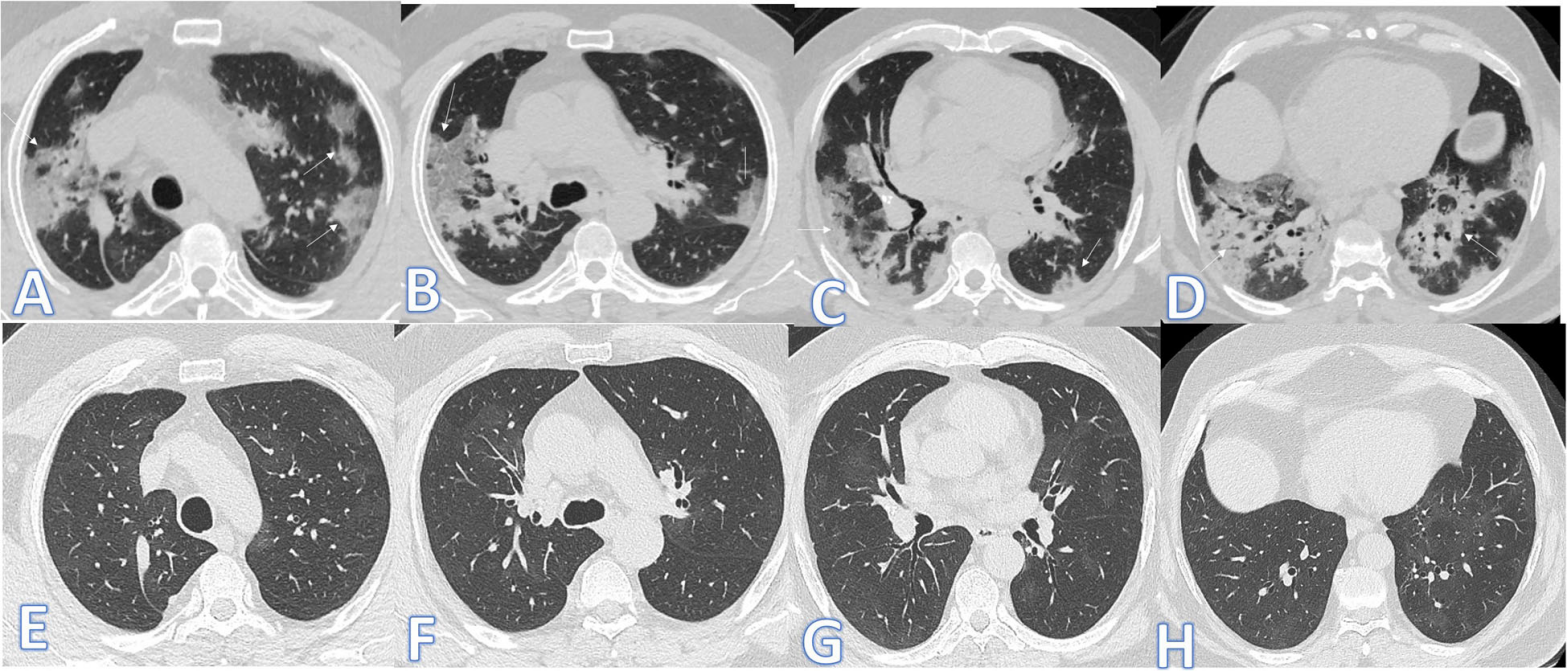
Twenty-five-year-old male: he has no previous medical illness: CT severity score 14; consolidation/crazy-paving score 12. No ICU admission; laboratory results show lymphopenia, high CRP, d-dimer, and serum ferritin. Steroid was given; length of stay during hospitalization is10 days. Peak CT during admission (a–d) showed bilateral central and peripheral consolidation patches mainly in the lower lobes with GGO and a crazy-paving pattern (arrows). Follow-up CT 2 months after discharge (e–h) showed total resolution of the previous lung changes
